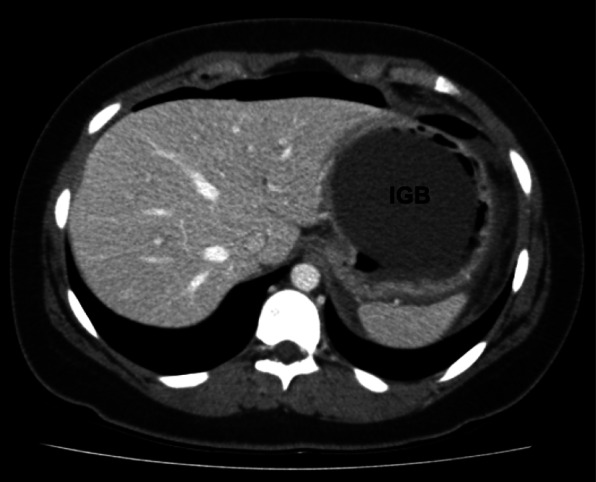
CT-scan of the upper abdomen with free abdominal air and indwelling intragastric balloon. IGB, intragastric balloon
